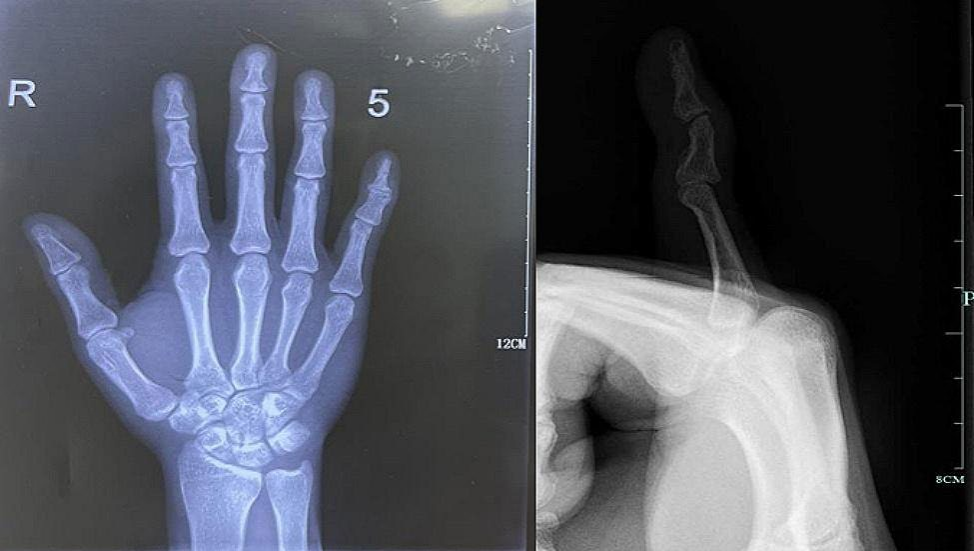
X-ray of the right hand presenting a malunion fracture block at the middle and distal phalanges of the right little finger
